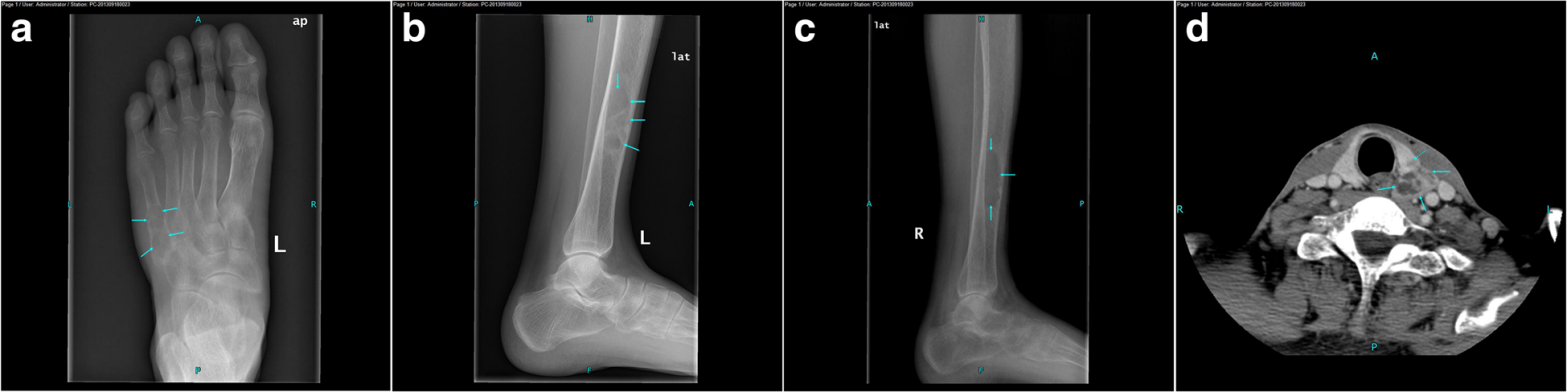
(a-c) Radiographs showing multiple mild but expansive osteolytic lesions in the bilateral distal tibia and left fifth metatarsal bone. (d) Axial CT scan of the neck showing a mass lesion measuring 2.7 × 1.4 cm on the left parathyroid (arrow) and a round lesion measuring 1.5 × 1.0 cm in the left thyroid lobe (dotted arrow)
